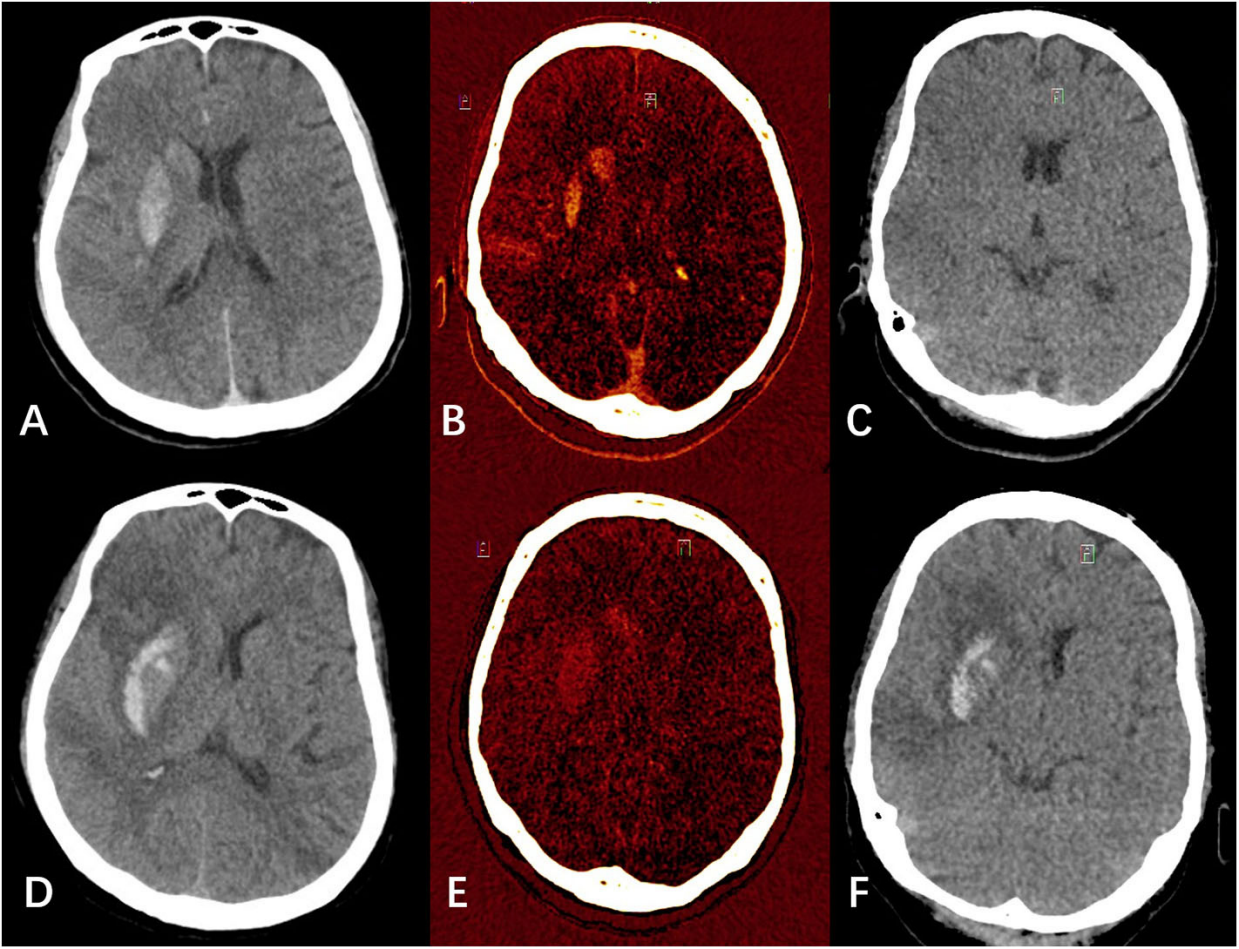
Examples of hemorrhagic transformation and contrast extravasation with iodine overlay map (IOM), virtual non-contrast (VNC), and mixed images. (A–C) were mixed image, IOM, and VNC, respectively from a patient's dual energy CT (DECT) immediately after endovascular thrombectomy (EVT). (A) showed hyperdensities in the right lentiform nucleus and caudate nucleus. In (B,C) combined, the hyperdensities were classified as pure iodine contrast. (D–F) were mixed image, IOM, and VNC, respectively from the same patient's DECT 24 h after EVT. (D) also showed hyperdensities in the right lentiform nucleus and caudate nucleus. In (E,F) combined, the hyperdensities were classified as hemorrhage with iodine contrast.
